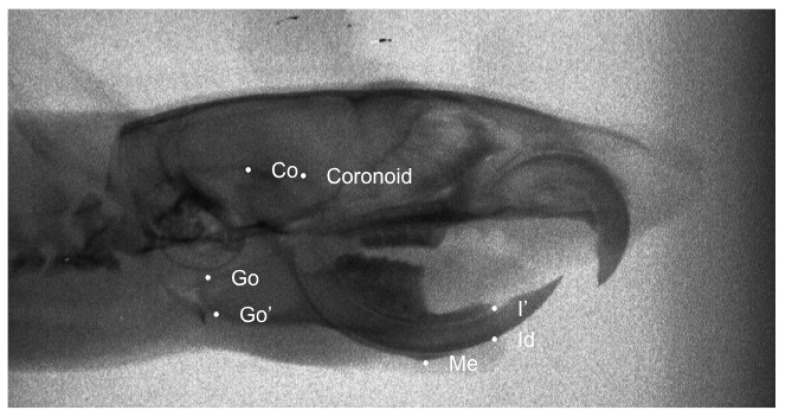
Traced X-ray for linear mesasurement. The landmarks we used are the most posterior-superior point on the mandibular condyle (Co), the most posterior point of the angular process of the mandible (Go), the point on the most inferior contour of the angular process of the mandible (Go’), the most posterosuperior point of condylar process (Coronoid), the most inferior and anterior point of the lower border of the mandible (Me), the most inferior and anterior point on the alveolar process of the mandible (Id) and the most anterior edge of the alveolar bone on the convexity of the lower incisor (I’).
